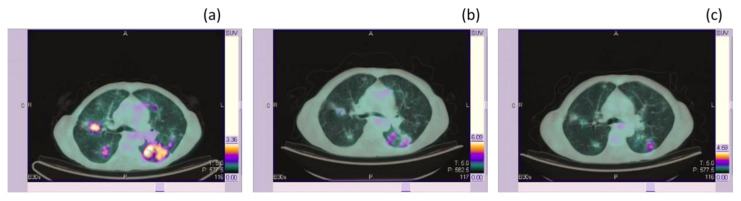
An example of early metabolic response after therapy with Erlotinib in a patient with no smoking history and lung adenocarcinoma. Marked [^18^F]FDG uptake is evident at baseline in bilateral lung nodules (SULpeak 7 in the right inferior pulmonary lobe) (a). First response (b) three months after therapy initiation highlights a marked reduction of [^18^F]FDG PET uptake in all lung nodules in the absence of a significant reduction in lesion size as evident in the coregistered CT (SULpeak 2 in the right inferior pulmonary lobe, resulting in a partial metabolic response according to the PERCIST criteria while the patient was classified as stable disease according to the RECIST criteria). Nine months after therapy, (c) the metabolic response was still clearly evident and was associated with a measurable reduction also in the lesion size (partial response based also on the RECIST criteria). SUL: Standardized uptake value corrected for the lean body mass; [^18^F]FDG: 2-deoxy-2-[^18^F]fluoro-D-glucose; PET: positron emission tomography; CT: computed tomography; RECIST: Response Evaluation Criteria in Solid Tumors.
